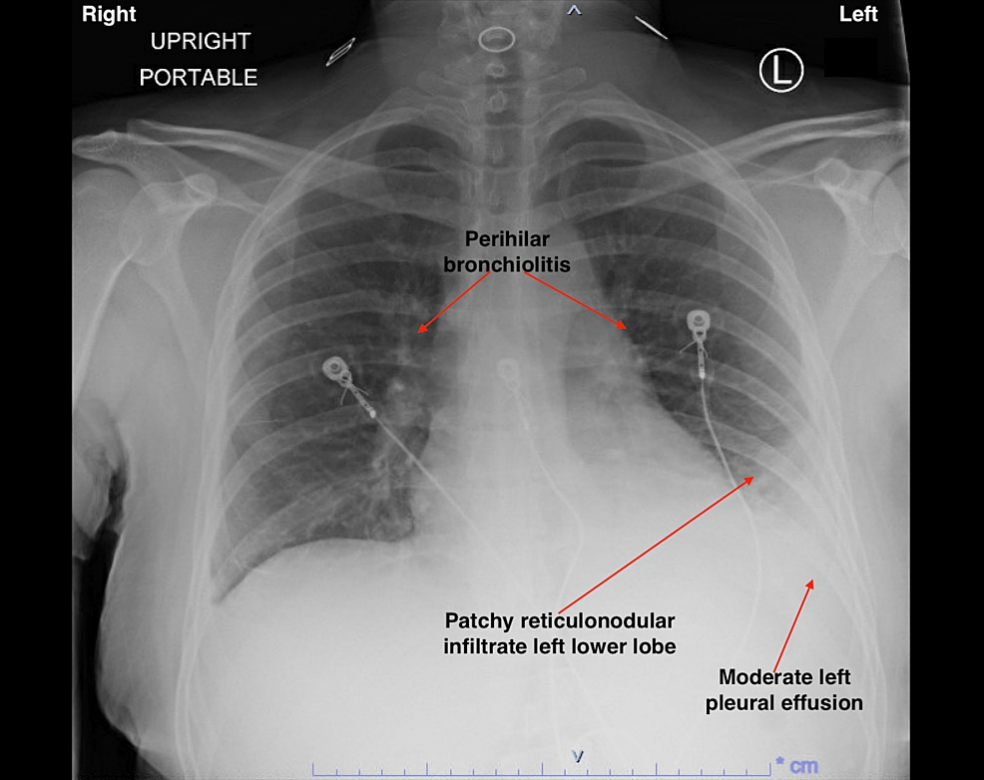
Chest X-ray on admission The image is showing a patchy reticulonodular infiltrate left lower lobe, moderate left pleural effusion, and perihilar bronchiolitis.
